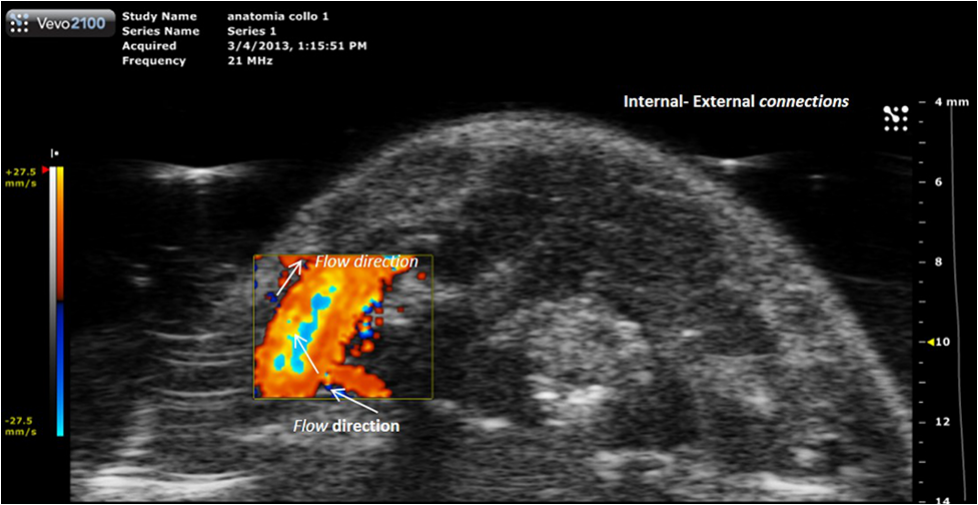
Eco-Color Doppler of vein collaterals. Collateral vein that connect external jugular vein and vertebral plexus. The flow is directed from vertebral plexus to the right external jugular vein.
